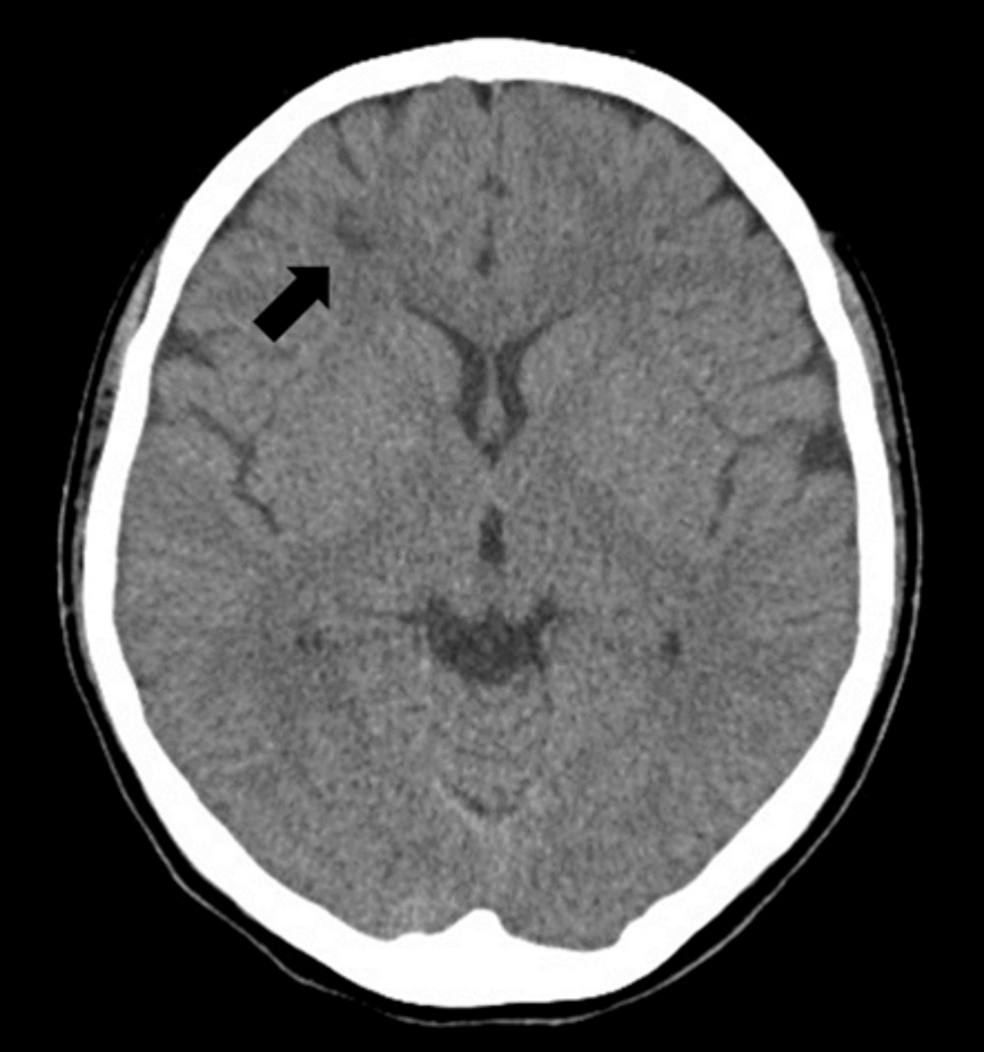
Computed tomography revealed two low-density areas in the right frontal lobe (black arrow).
